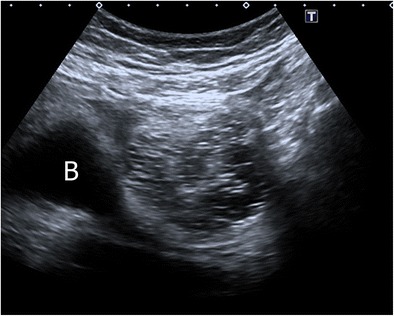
Transverse sonogram of a 51-year-old female with mature cystic teratoma. Echogenic lines and dots, making the dot-dash sign, are seen in a left adnexal mass regarding hair arranged in different orientations. *B* bladder
